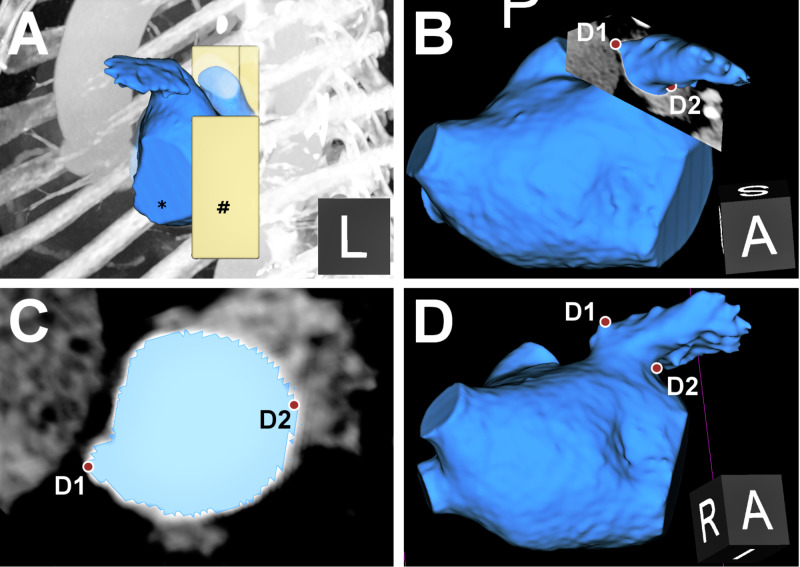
CT analysis and determination of personalized fluoroscopic angles (A) The left atrial appendage (*, blue) and personalized stand (#, yellow) are segmented from CT and reconstructed into a 3D model. The model is overlaid on a left lateral maximum intensity projection. A customized CT slice (multiplanar reconstruction) is cut across the target Watchman landing zone. Through inspection of 3D CT (B) and custom slice (C), the extent of the maximum LAA landing zone diameter is measured and marked with two fiducials (D1 and D2). (D) A 3D plane of view is selected wherein both fiducials are visible precisely at the borders of the LAA silhouette. Personalized fluoroscopic angles to replicate this view can be computed. When personalized angles are reproduced on fluoroscopy, an identical cardiac silhouette and landing zone to the one marked on CT should be observable. An analogous process was used to select personalized angles for maximum LAA length. 3D, three-dimensional; LAA, left atrial appendage.
